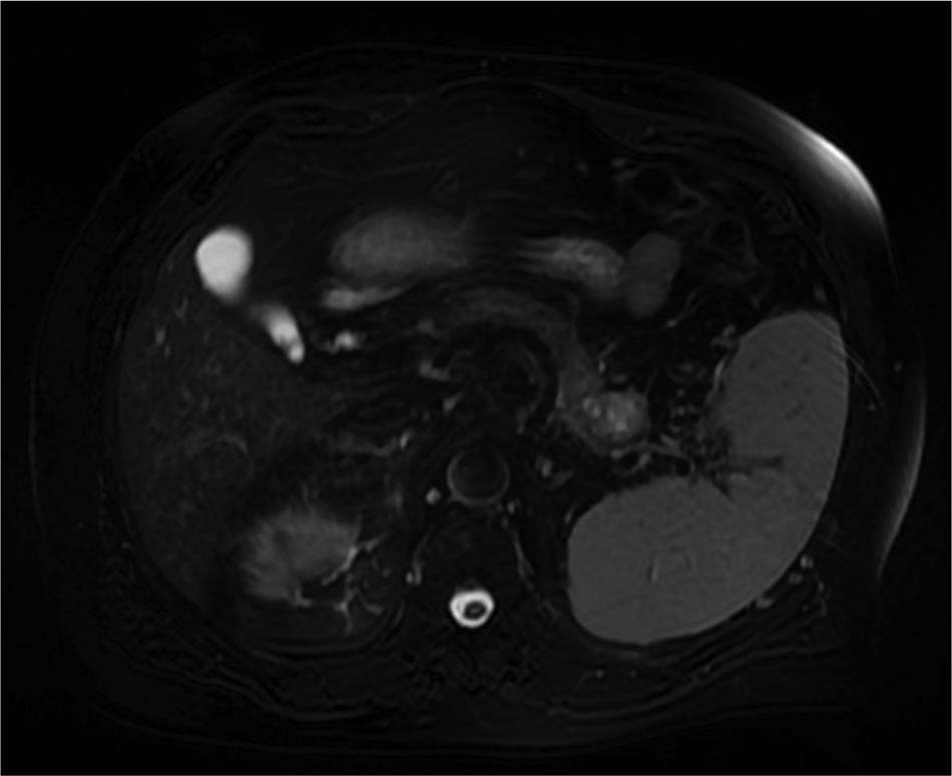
MRI (venous phase) shows a 2.6 cm lesion in the pancreatic tail, exhibiting heterogeneous *T*_2_ signal intensity with areas of high signal but lacking diffusion restriction.
